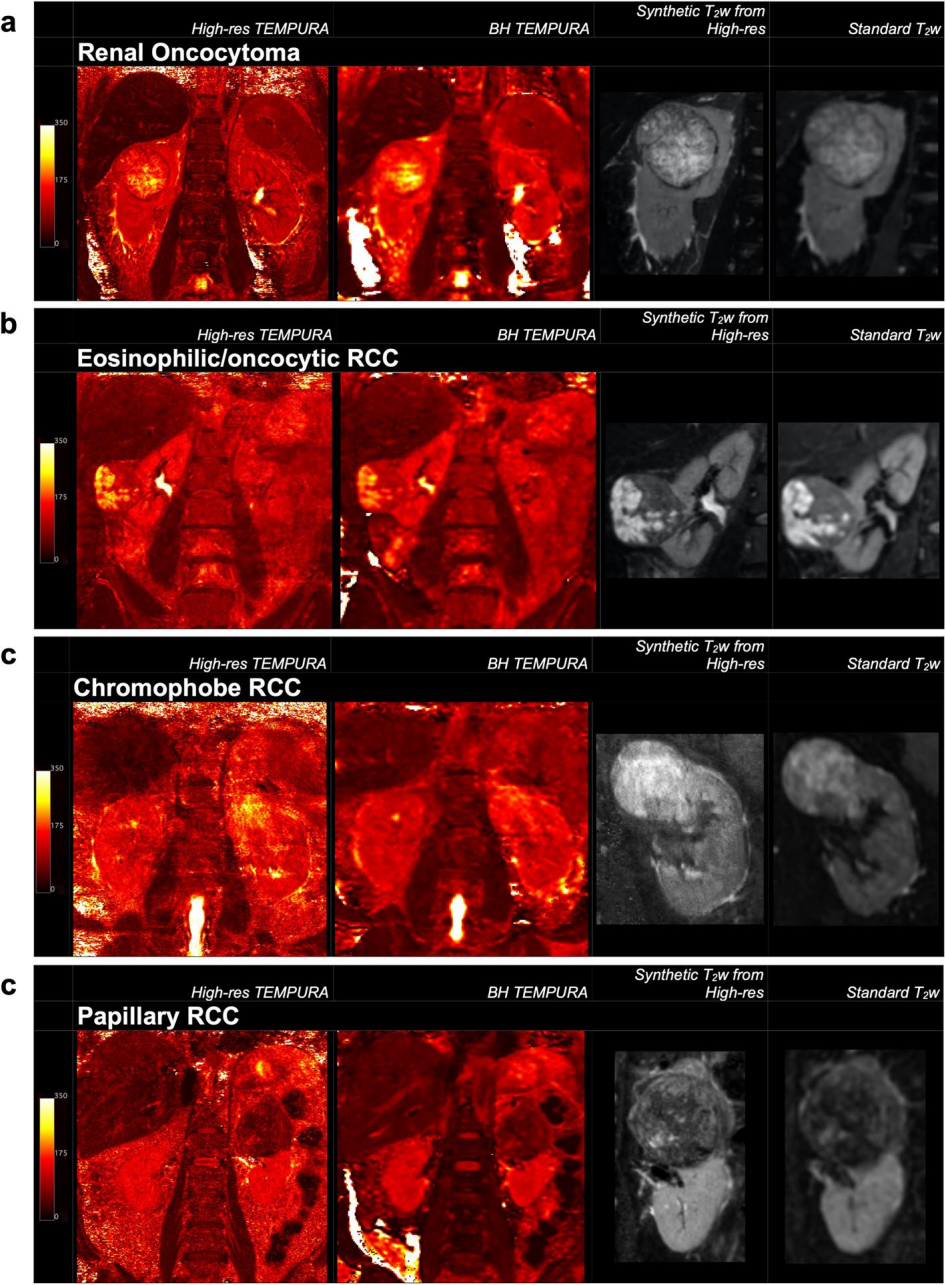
Representative T2 maps from the patient cohort. High-resolution TEMPURA, BH TEMPURA, synthetic T2-weighted from High-res TEMPURA and standard T2-weighted from: (a) renal oncocytoma; (b) eosinophilic/oncocytic RCC; (c) chromophobe RCC; and (d) papillary RCC. *TEMPURA* T2 mapping methods based on echo merging and using *k*-t undersampling and reduced flip angles; *RCC* Renal cell carcinoma
